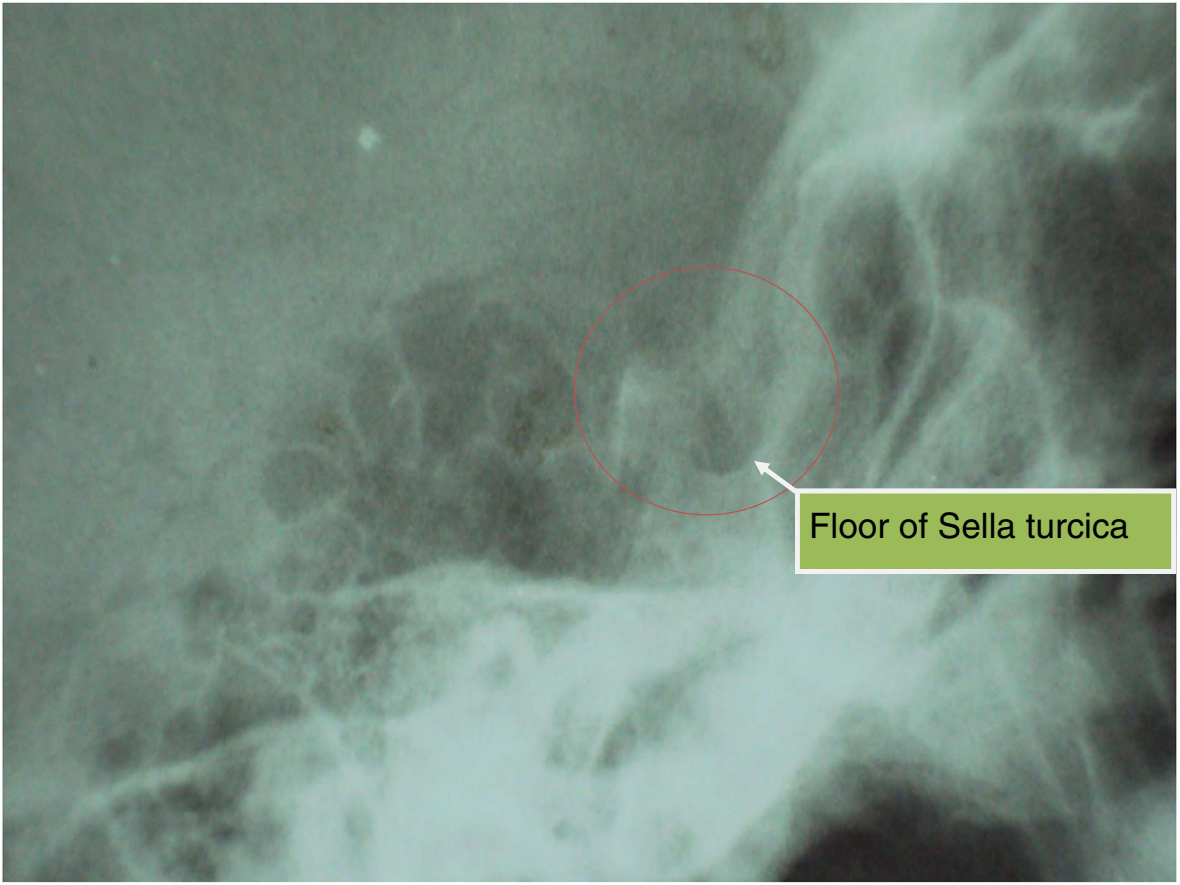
Sella turcica X-ray*(lateral view). Legend: No evidence of mass lesion seen, symmetric floor with no erosion or `double floor` sign.
